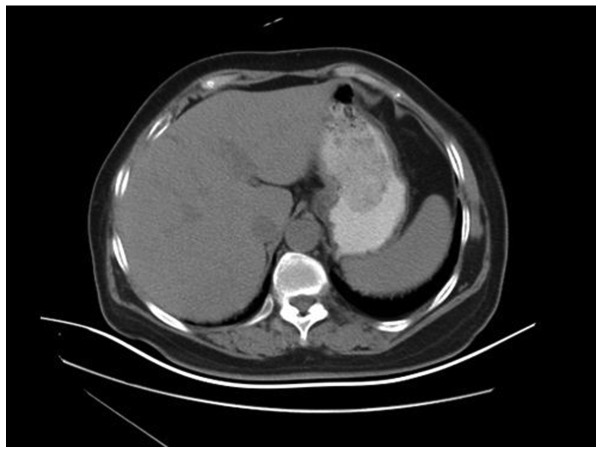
Pre-operative abdominal CT demonstrating a 5.5×5.3-cm solid mass located in the posterior wall of the stomach. Enhanced CT shows peripheral enhancement, low-density areas and a gas shadow. CT, computed tomography.
